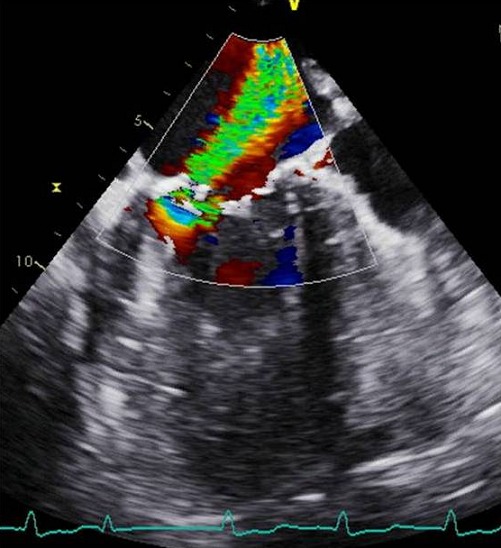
Coupe 3 cavités en échographie transoesophagienne (ETO) au Doppler couleur montrant une fuite mitrale importante à travers l’anévrysme mycotique
